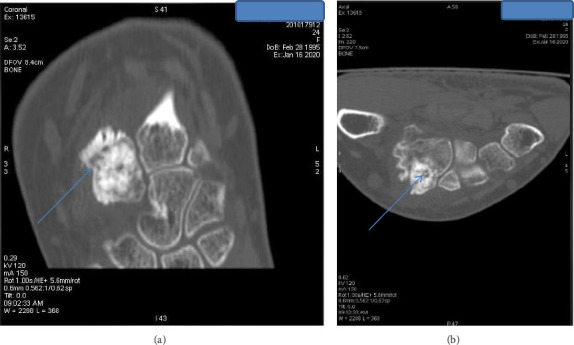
The coronal (a) and axial (b) computed tomography show an intra-articular nidus at the trapezoidal bone surrounded by sclerotic lesions at the trapezoidal and the base of the second metacarpal bones.
